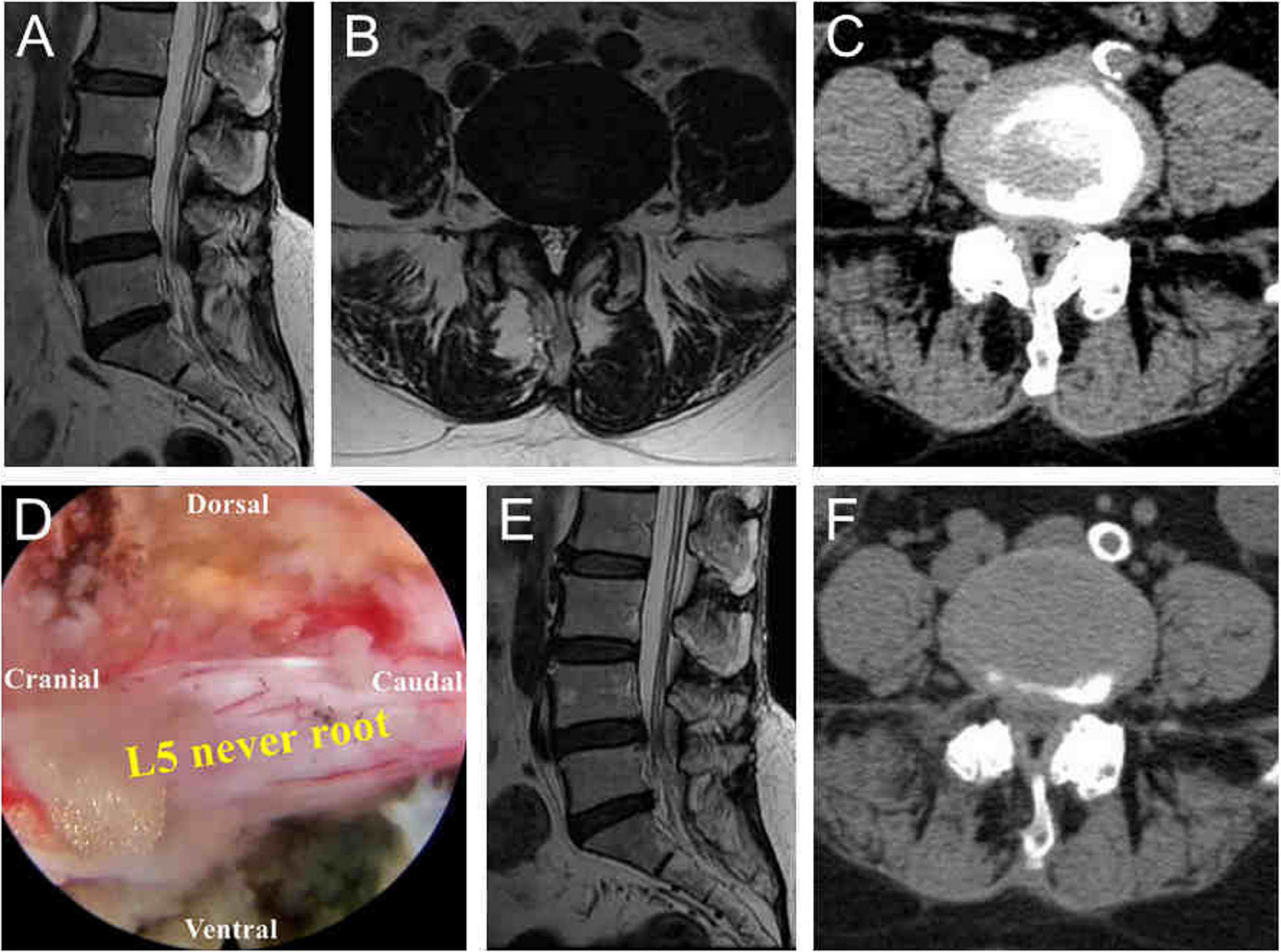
A case with lateral recess stenosis treated by the visualized reamed foraminoplasty technique. Preoperative MRI (a, b) and CT (c) of a 60-year-old woman with right leg radiating pain showed lateral recess stenosis and disc herniation of L4/5. The L5 nerve root was fully released after decompression (d). Postoperative MRI and CT showed that the lateral recess was enlarged, and the dorsal and ventral regions of the L5 nerve root were completely decompressed (e, f)
